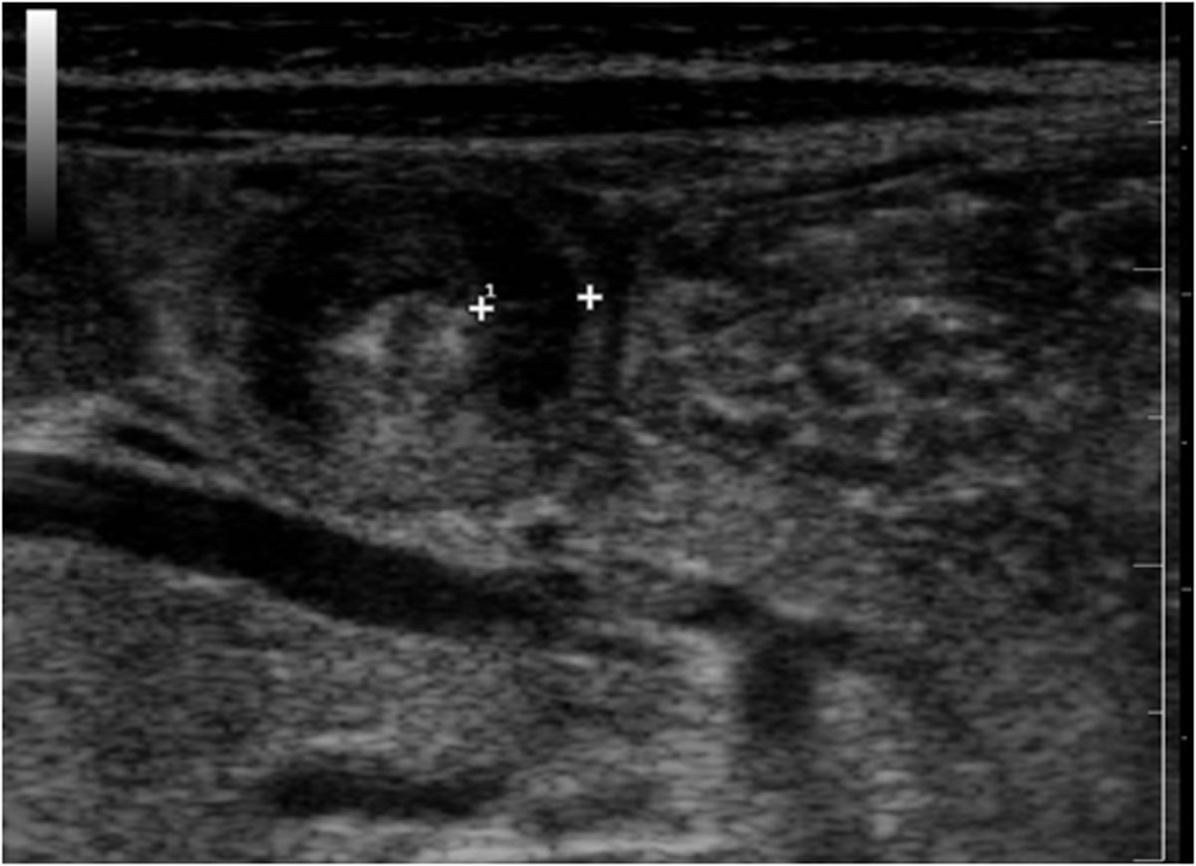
Ultrasound features of hypertrophic pyloric stenosis. On the cross scanning with the infant in the supine position is visualized the “donut sign” consists of a prominent anechoic rim of thickened muscle, and an echogenic center of mucosa and submucosa .
